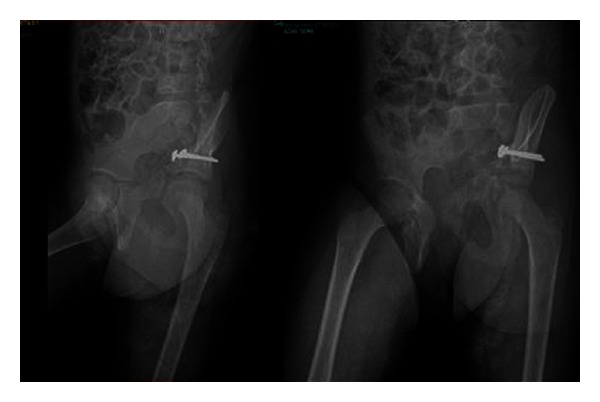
Early postoperative pelvis AP X-ray.
